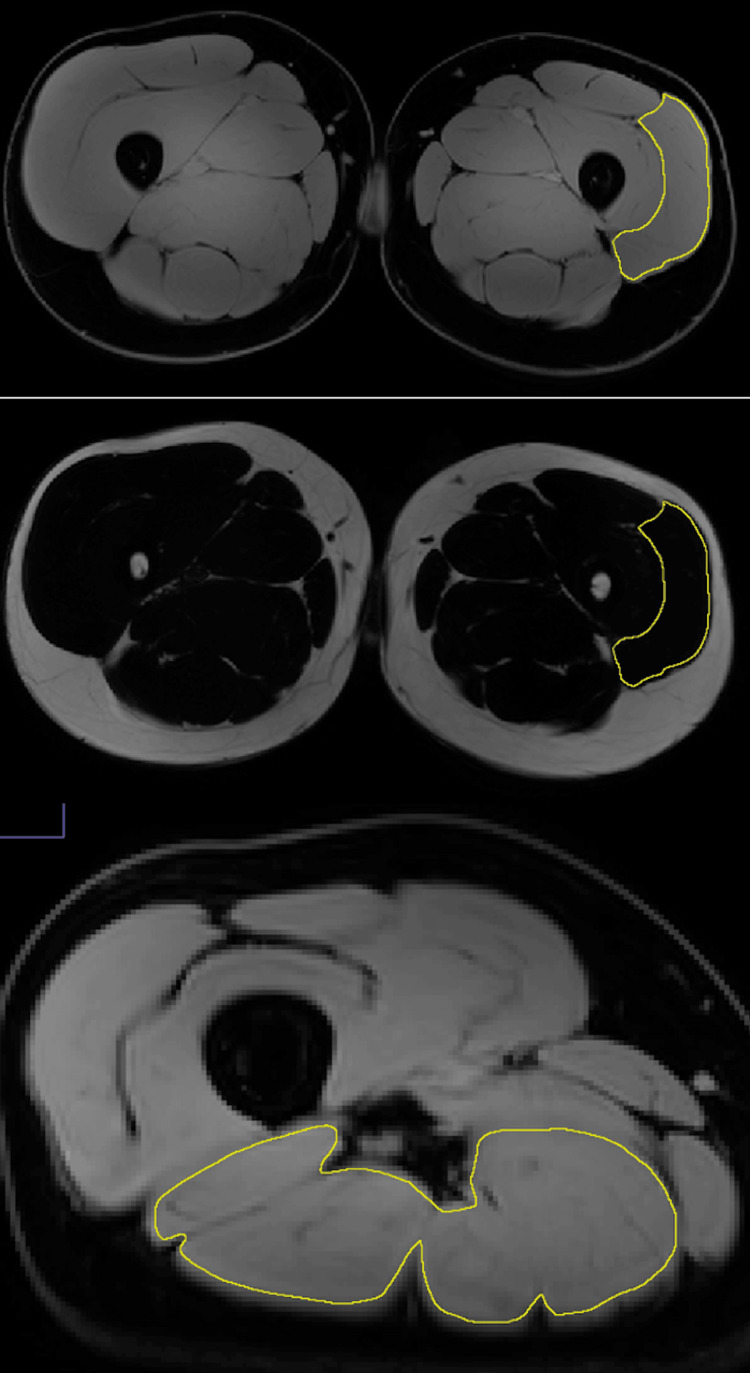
Magnetic resonance images with representative cuts demonstrating (A) ROI drawn on water phase image, (B) ROI copied to fat phase image, and (C) ROI excluding visible fascia and fat ROI: region of interest
